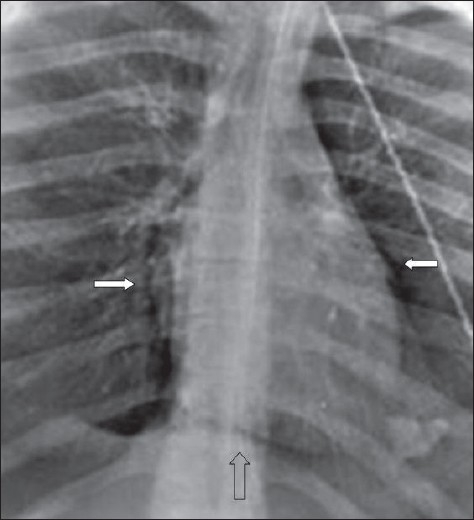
Chest x-ray findings of a pneumopericardium shown as a lucent line around the heart extending up to the main pulmonary arteries (solid white arrows). Air may accumulate inferior to the cardiac shadow, which crosses the midline above the diaphragm, which is said to be diagnostic for pneumopericardium, the so-called continuous diaphragm sign (hollow arrow)
